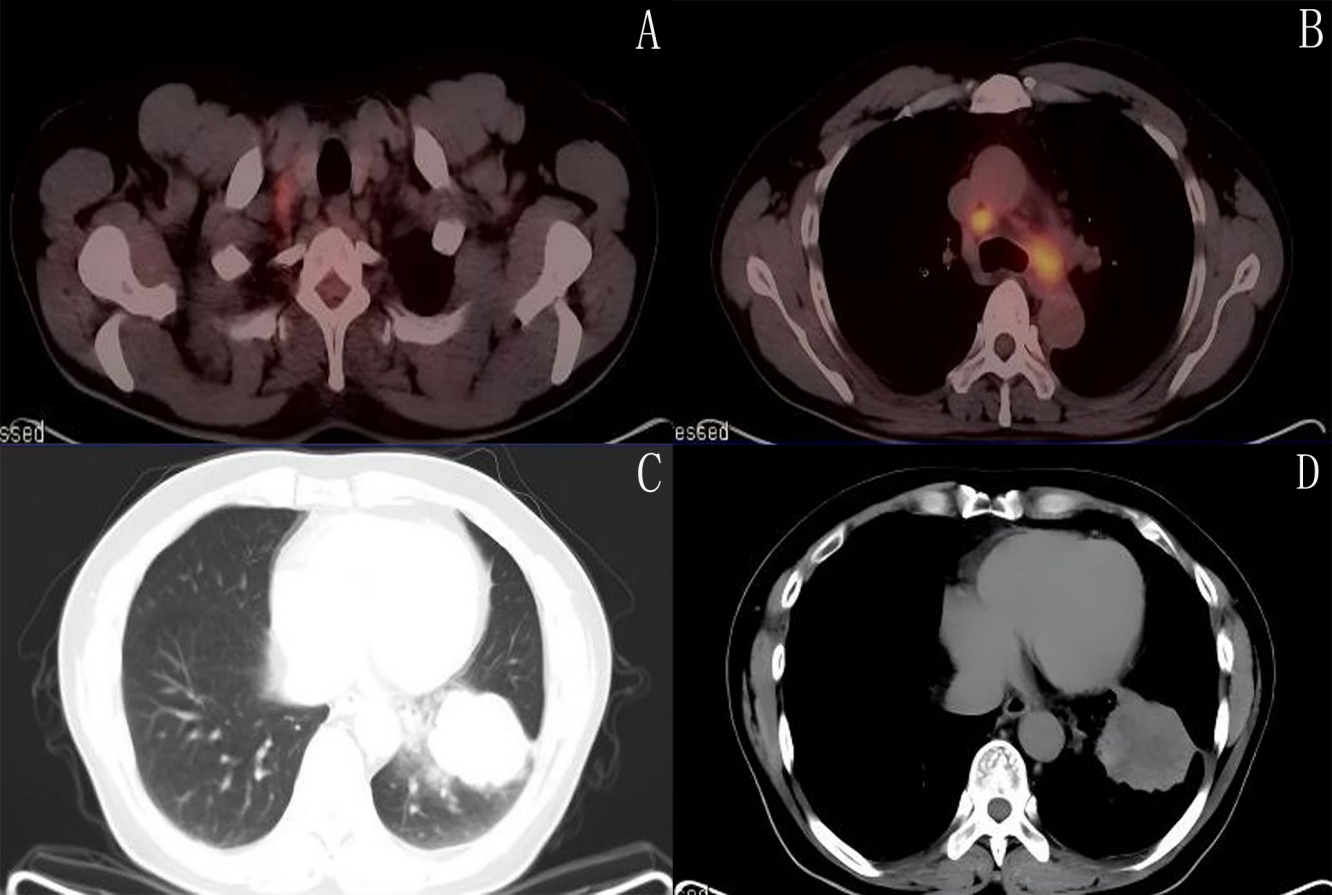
Baseline Image (PET-CT on March 25, 2021). (A) Right supraclavicular lymph node metastasis. (B) Mediastinal and left hilar lymph node metastasis. (C) Pulmonary window:solid mass in the left lower lobe of the lung. (D) Diaphragmatic window: solid mass in the left lower lobe of the lung.
